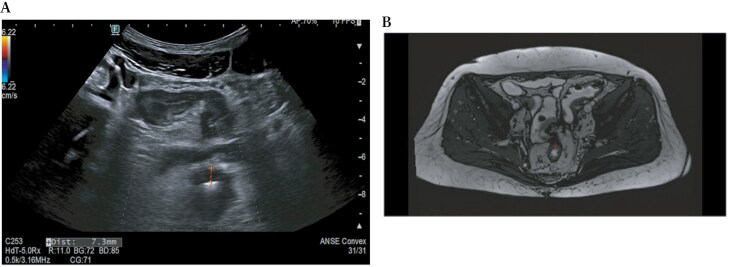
Intestinal ultrasound (A) and magnetic resonance enterography (B) show a wall thickening of the rectum up to 7.3 mm (red dotted lines).
